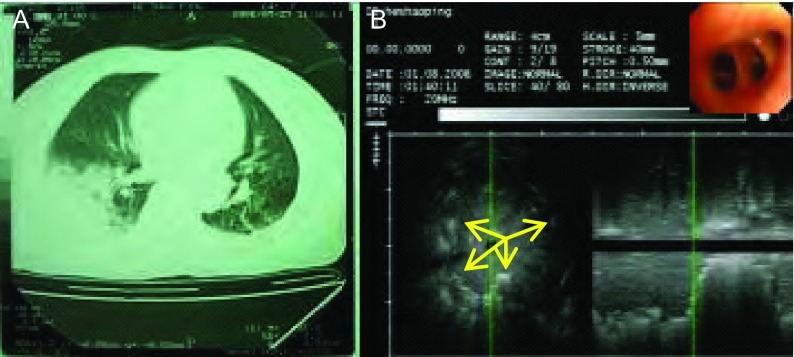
女，63岁，发热伴咳嗽咳痰1周，抗生素治疗1周后病灶明显吸收，肺炎。A：CT见右下肺实变；B：支气管镜下未见异常，右下叶外基底段行支气管内超声检查见病灶内部回声均匀，边界部分不清，部分呈锯齿状，支气管充气征位于周边，近似同心圆状规则分布（箭头）。 Female, 63 years old, fever complicated with cough and expectoration of sputum for 1 week, after 1 week of antibiotic therapy, the lesion was absorbed, diagnosed pneumonia. A: CT shows consolidation at the right lower lobe; B: No abnormality found by bronchoscope, endobronchial ultrasound in the lateral basal segment showed homogeneous echo in the lesion, the border was fuzzy, partially serrated, air bronchogram found at periphery part, distributed regularly at the shape similar to concentric circles (at the arrow tip).
